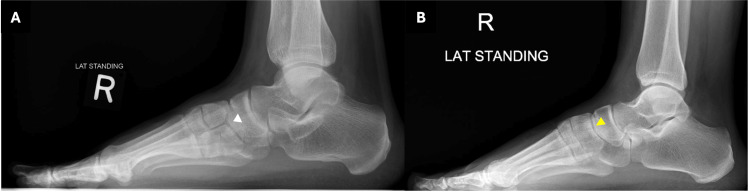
Lateral weight-bearing radiograph with (A) bipartite medial cuneiform (white arrowhead) compared to a (B) normal medial cuneiform in a different patient (yellow arrowhead).
